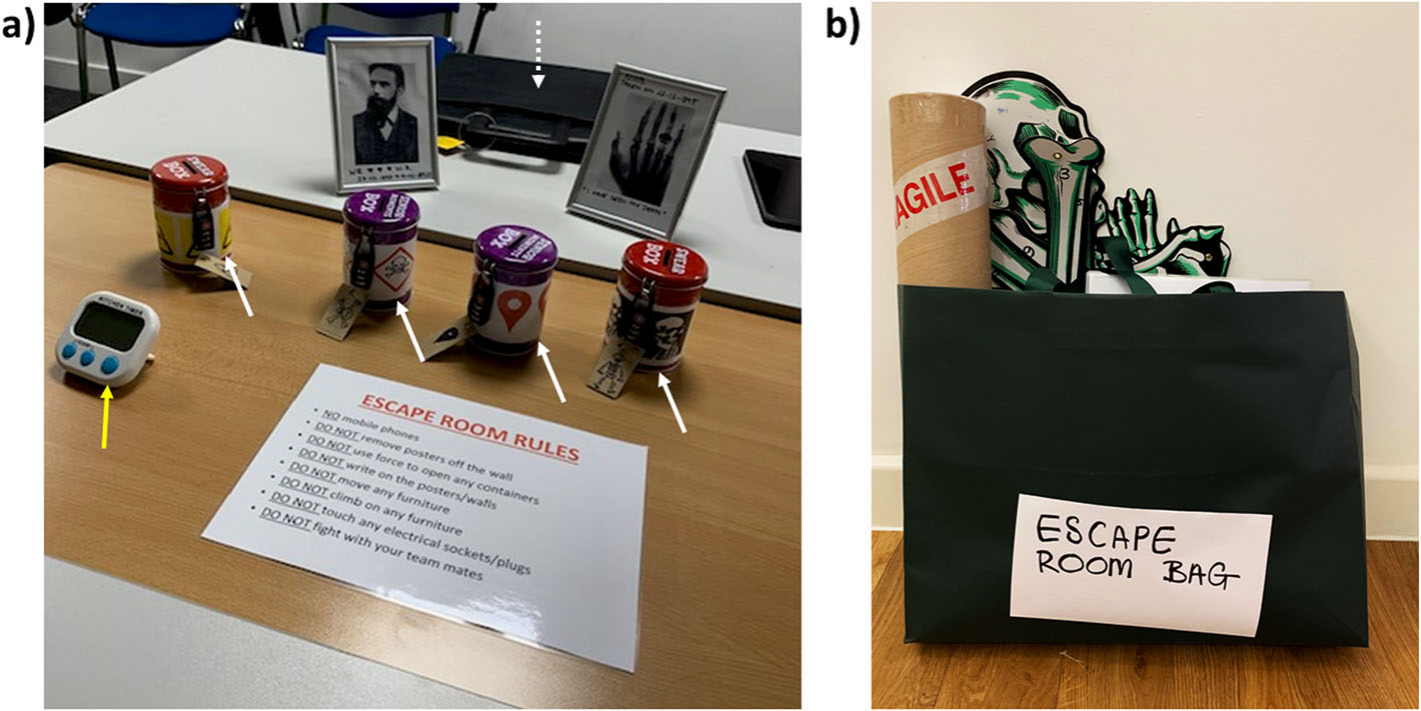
Equipment in the escape room. a The equipment on the central desk included a copy of the escape room rules, the kitchen timer to time the participants (yellow arrow), the four tin containers (white arrow) which were each locked with a three-number combination lock and contained a note for the final crossword puzzle, and clues on how to unlock the briefcase containing the puzzle (dashed white arrow). Photo frames containing images of Roentgen and an early example of a hand radiograph were not part of the escape room puzzles, and only placed on the table for decoration. b All the equipment was easily transportable between teaching sites within a single carry bag
